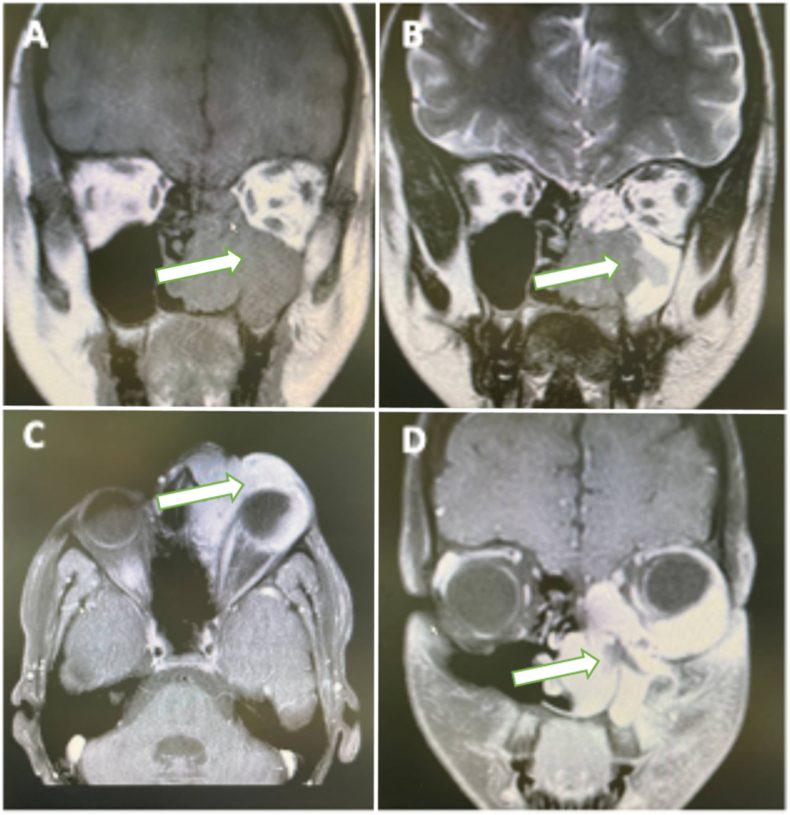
Coronal T1-weighted (A) and T2-weighted (B) MRI of paranasal sinuses showing an infiltrative mass in the left ethmoidal and maxillary sinuses extending to the right ethmoidal sinus and inferior surface of the left orbit. T1-weighted orbital MRI with gadolinium enhancement, axial (C) and coronal (D) view display a significant enhancement.
